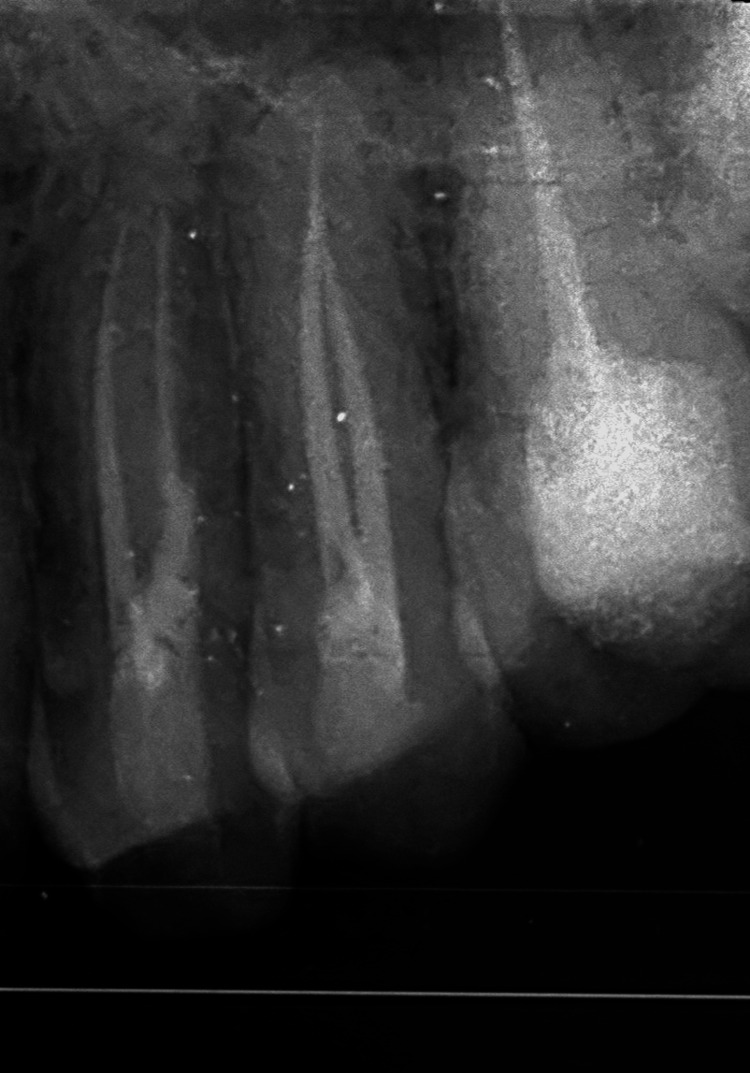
Postoperative radiograph of obturation and post-endodontic restoration of 24 and 25 Postoperative radiograph showing obturation and post-endodontic restoration of 24 and 25
